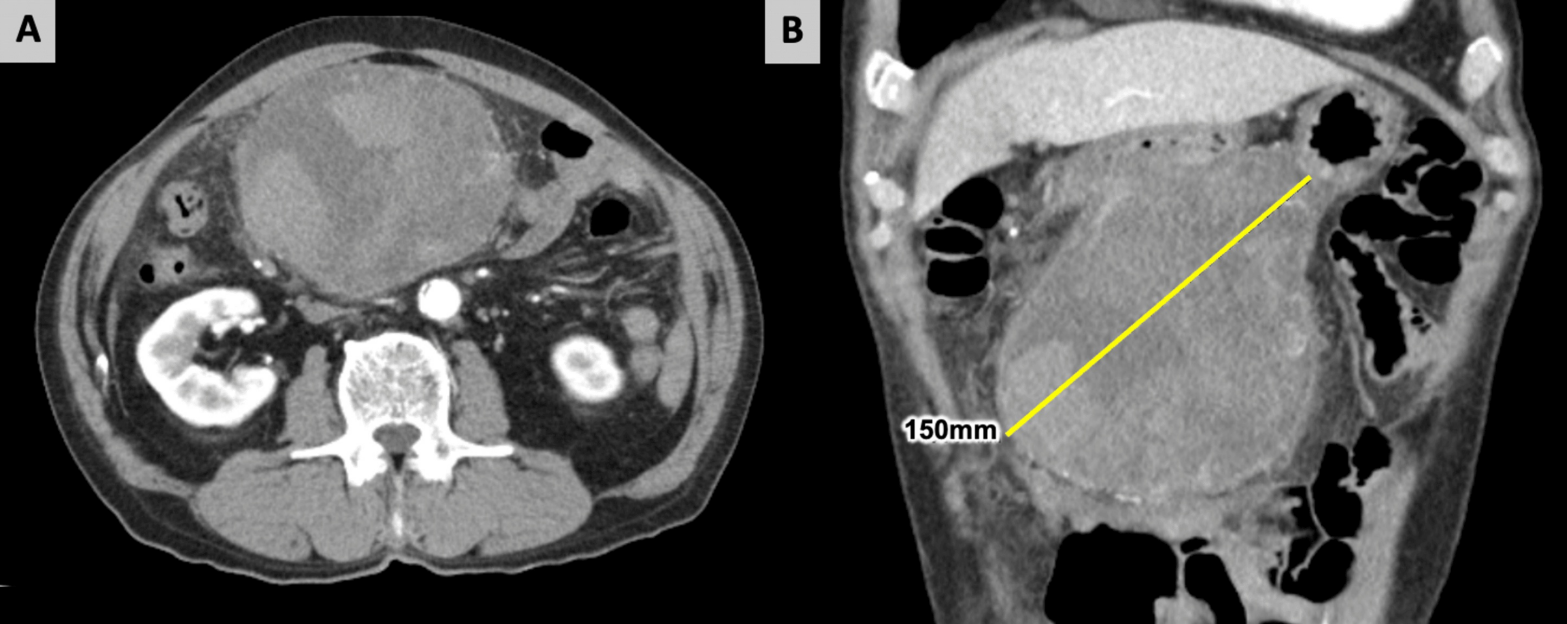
Contrast-enhanced abdominal CT on admission. (A) The axial view showed a huge mass consisting of heterogeneously enhanced solid and giant cystic lesions. (B) The coronal view showed a 100×150 mm mass. CT: computed tomography
